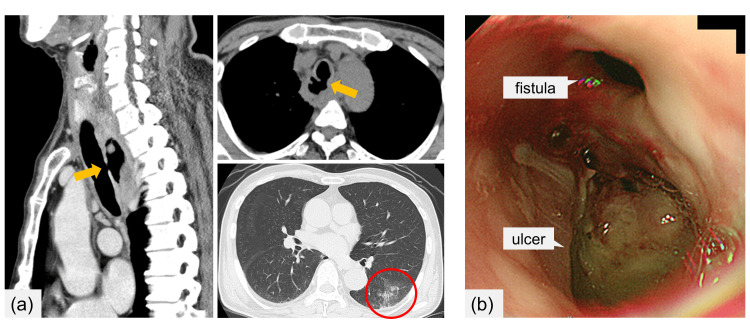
Computed tomography (CT) image and upper gastrointestinal endoscopy findings upon admission (a) CT image depicted a tracheoesophageal fistula measuring 5 mm in diameter and 1 cm in length, alongside aspiration pneumonia. (b) Upper gastrointestinal endoscopy findings revealed an ulcerous lesion and a fistulous connection to the trachea within the cervical esophagus.
